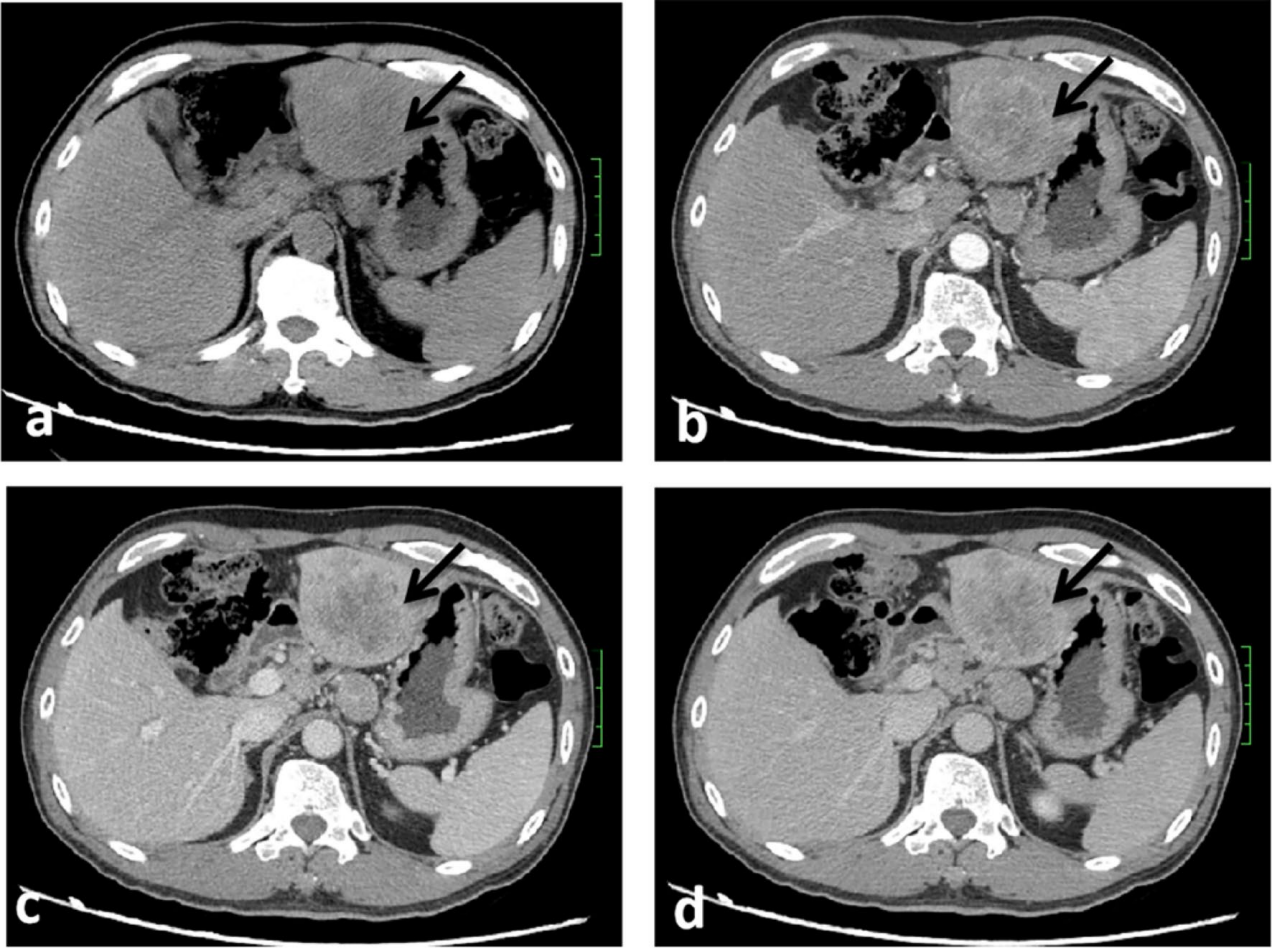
CT images in a 48-year-old HBV-positive man with ICC confirmed by surgical resection, AFP 3.79 ng/ml, CA19-9 163.6 U/ml, and its enhancement pattern was categorised as type IV. Precontrast image (a) showed a solitary tumor (arrow) in the left lobe of the liver; arterial phase (b) showed heterogeneous hyperenhancement; portal phase (c) and delayed phase (d) showed hypoenhancement.
